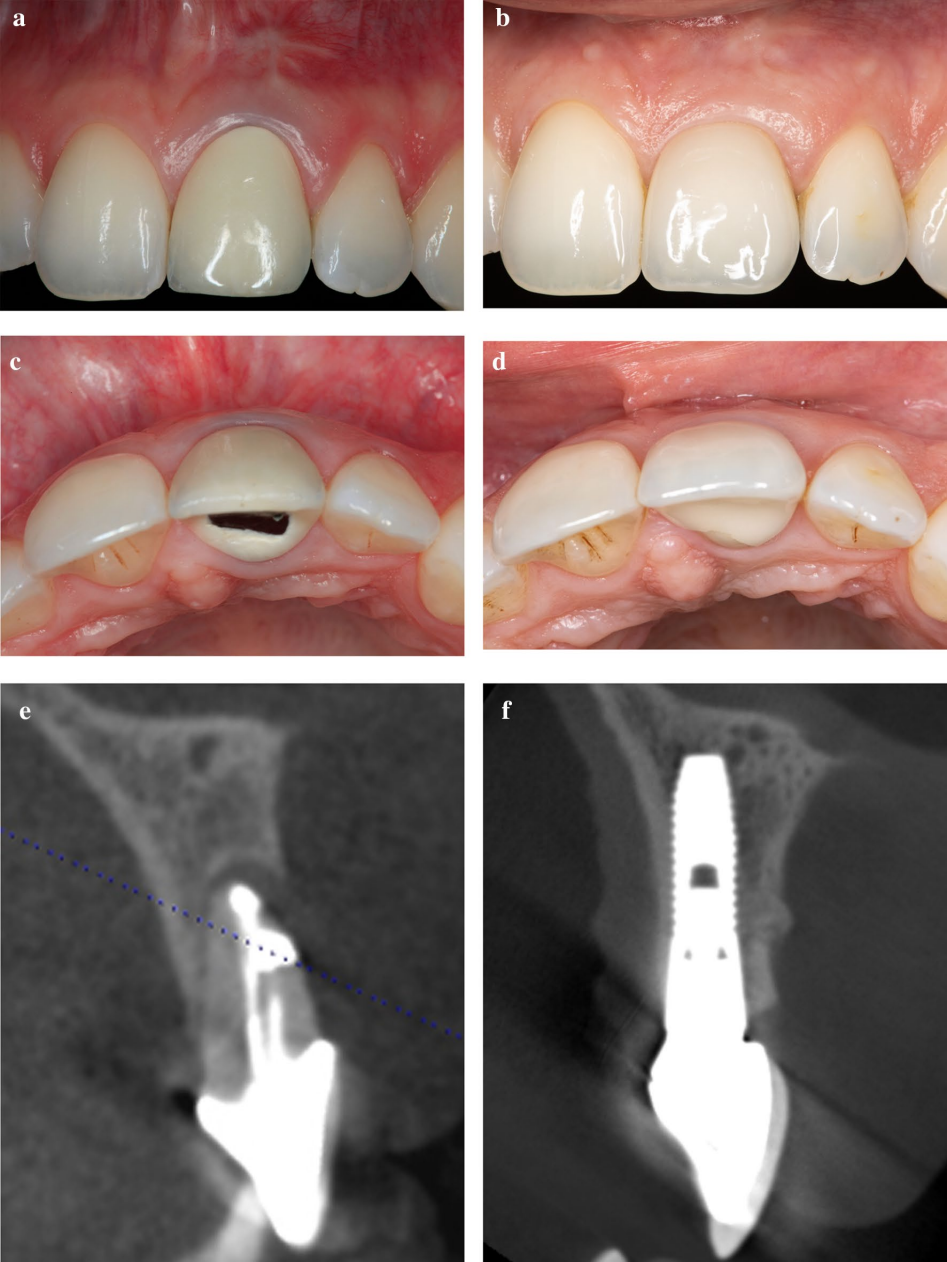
IIP in the presence of total buccal bone loss with flapless grafting with autogenous bone and connective tissue grafting in tunnel technique. a Thin mucogingival biotype and loss of buccal bundle bone followed by apicoectomy and vertical root fracture. b Healthy and thick peri-implant mucosa and increased soft tissue level 6 years after IIP and facial defect grafting with autogenous bone chips and connective tissue graft. c Occlusal view of the initial clinical situation with a thin and natural facial mucosa. d Six years after IIP and facial defect grafting with autogenous bone chips and connective tissue graft the facial alveolar contour presents naturally. e Preoperative CB-CT showing two root canal treatments, 2 retrograde fillings followed by an apicoectomy and the total loss of the buccal bone wall. f A CB-CT at 6y-follow-up examination shows the full reconstruction of the buccal bone wall close to the level of the implant shoulder
